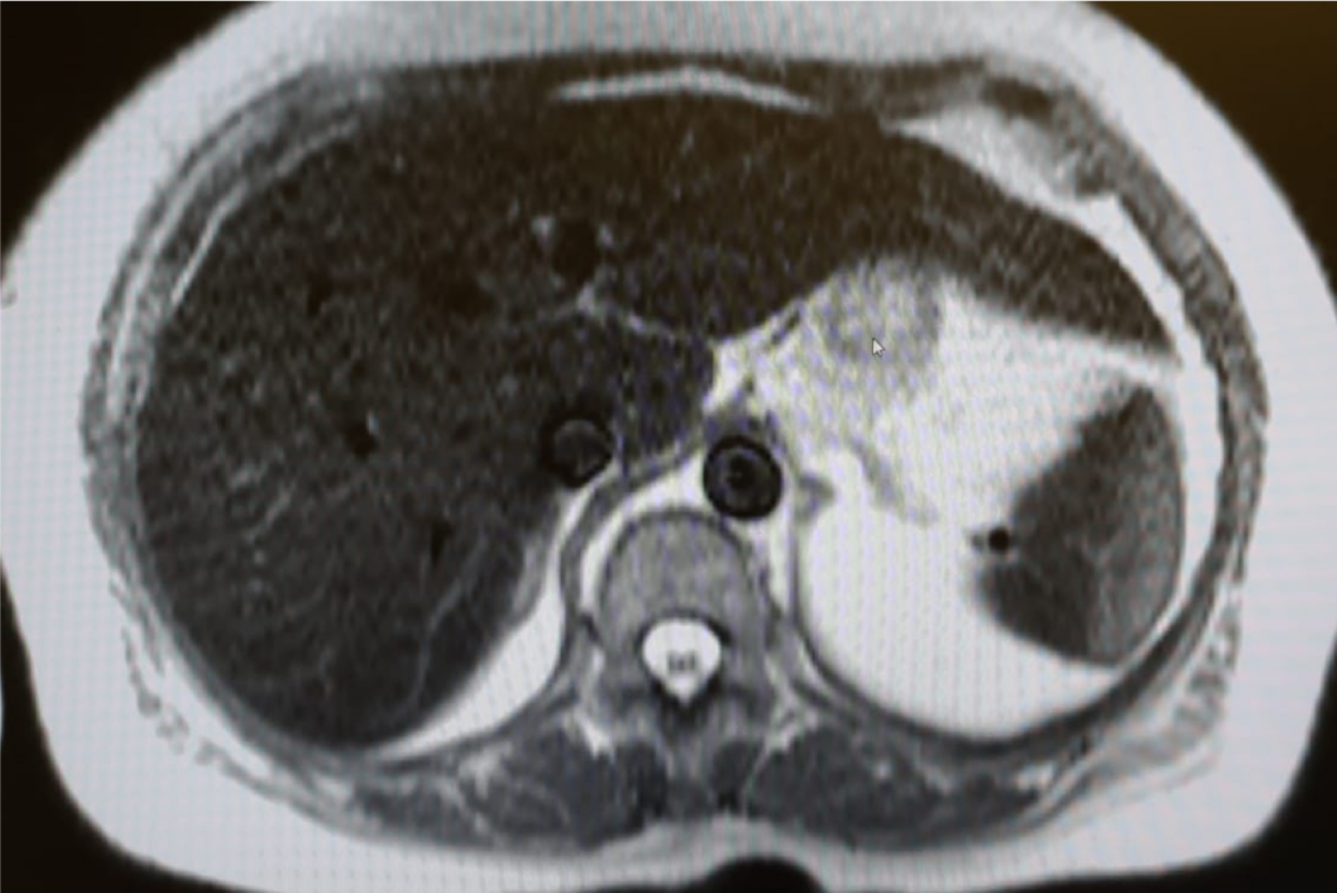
MRI T2* showing decreased liver signal intensity suggestive of iron overload; calculated LIC is 5.3 mg/g of dry liver weight, which proved mild iron overload. LIC, liver iron concentration
